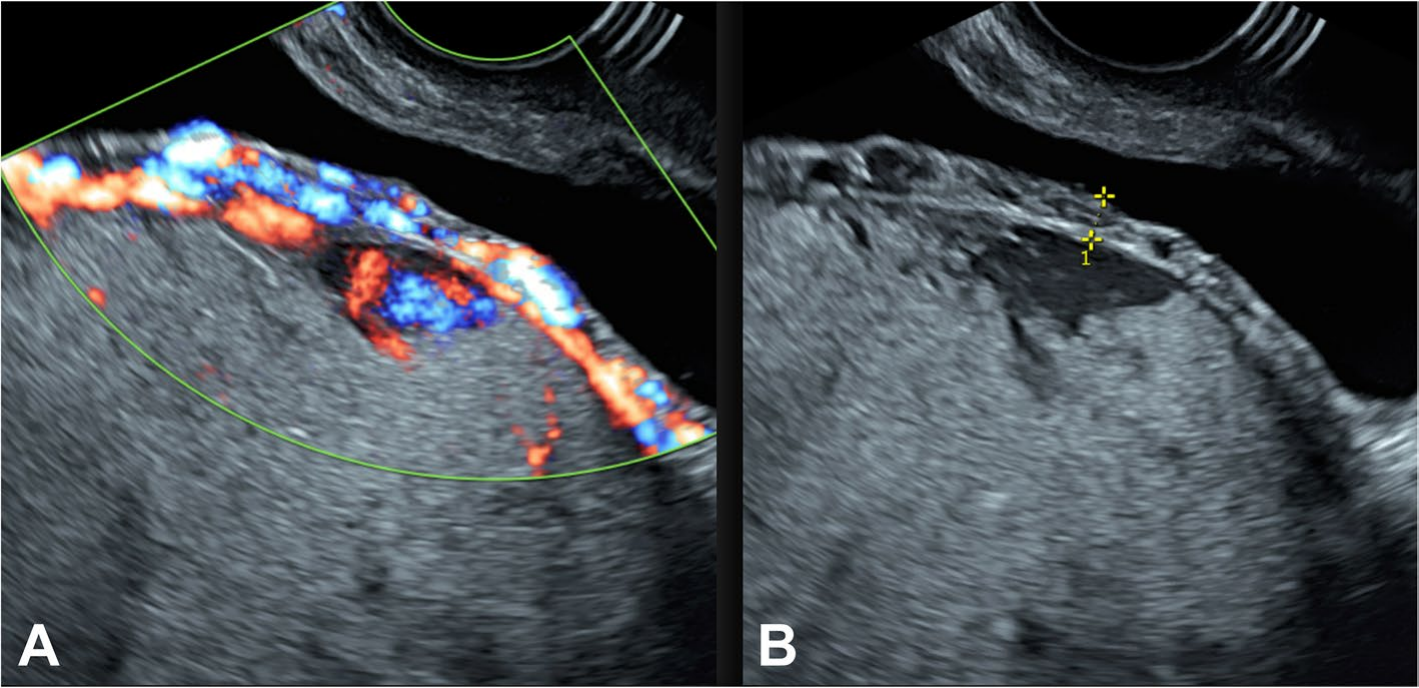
US and Doppler US study in PAS reveals the presence of abnormal placental lacunae, interruption of the bladder wall, myometrial thinning, and uterovesical hypervascularity
